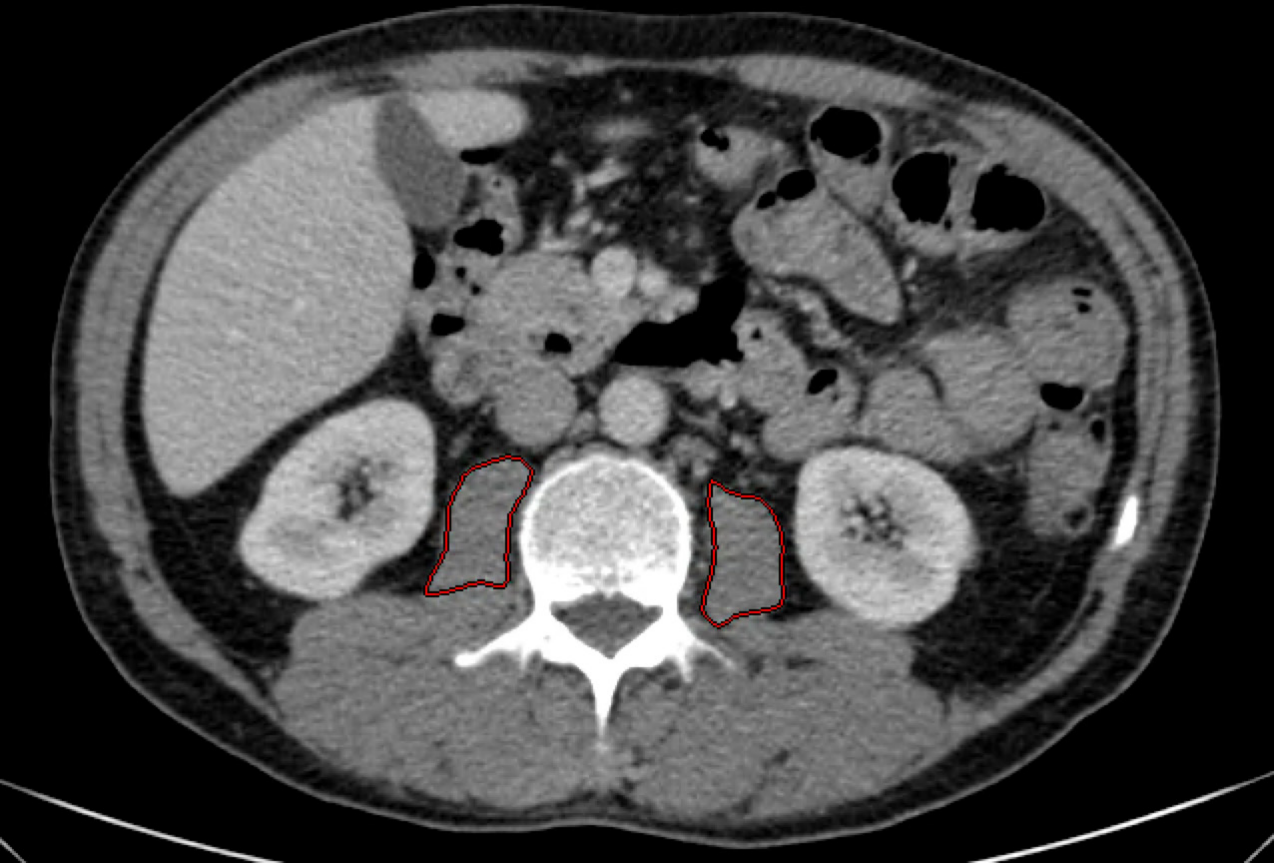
Radiological measurement of PMA. Figure 1 demonstrates the assessment of bilateral psoas muscle cross‐sectional areas through manual delineation of their contours on an axial CT scan. The combined area of both muscles, normalized to BSA, was utilized to derive the PMI. Abbreviations: BSA, body surface area; CT, computed tomography; PMA, psoas muscle area; PMI, psoas muscle area index; Q, quartile.
